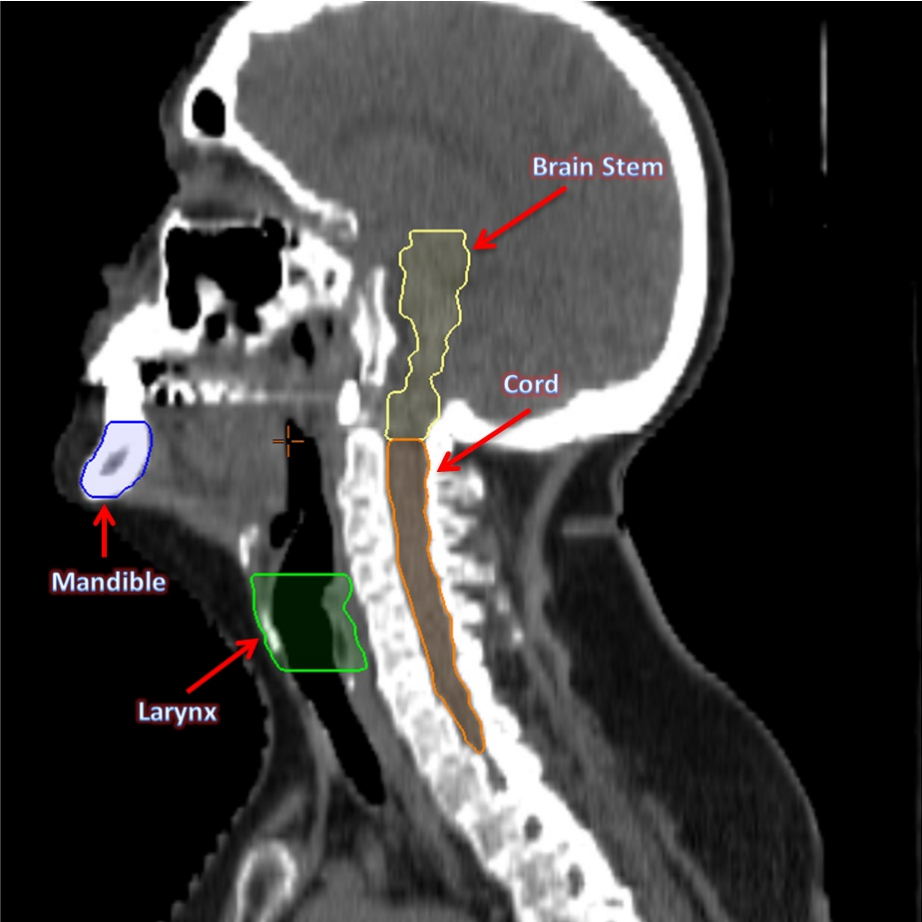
Sagittal view of the original head and neck CT image with the associated RT structures brain stem, cord, larynx, and mandible.
